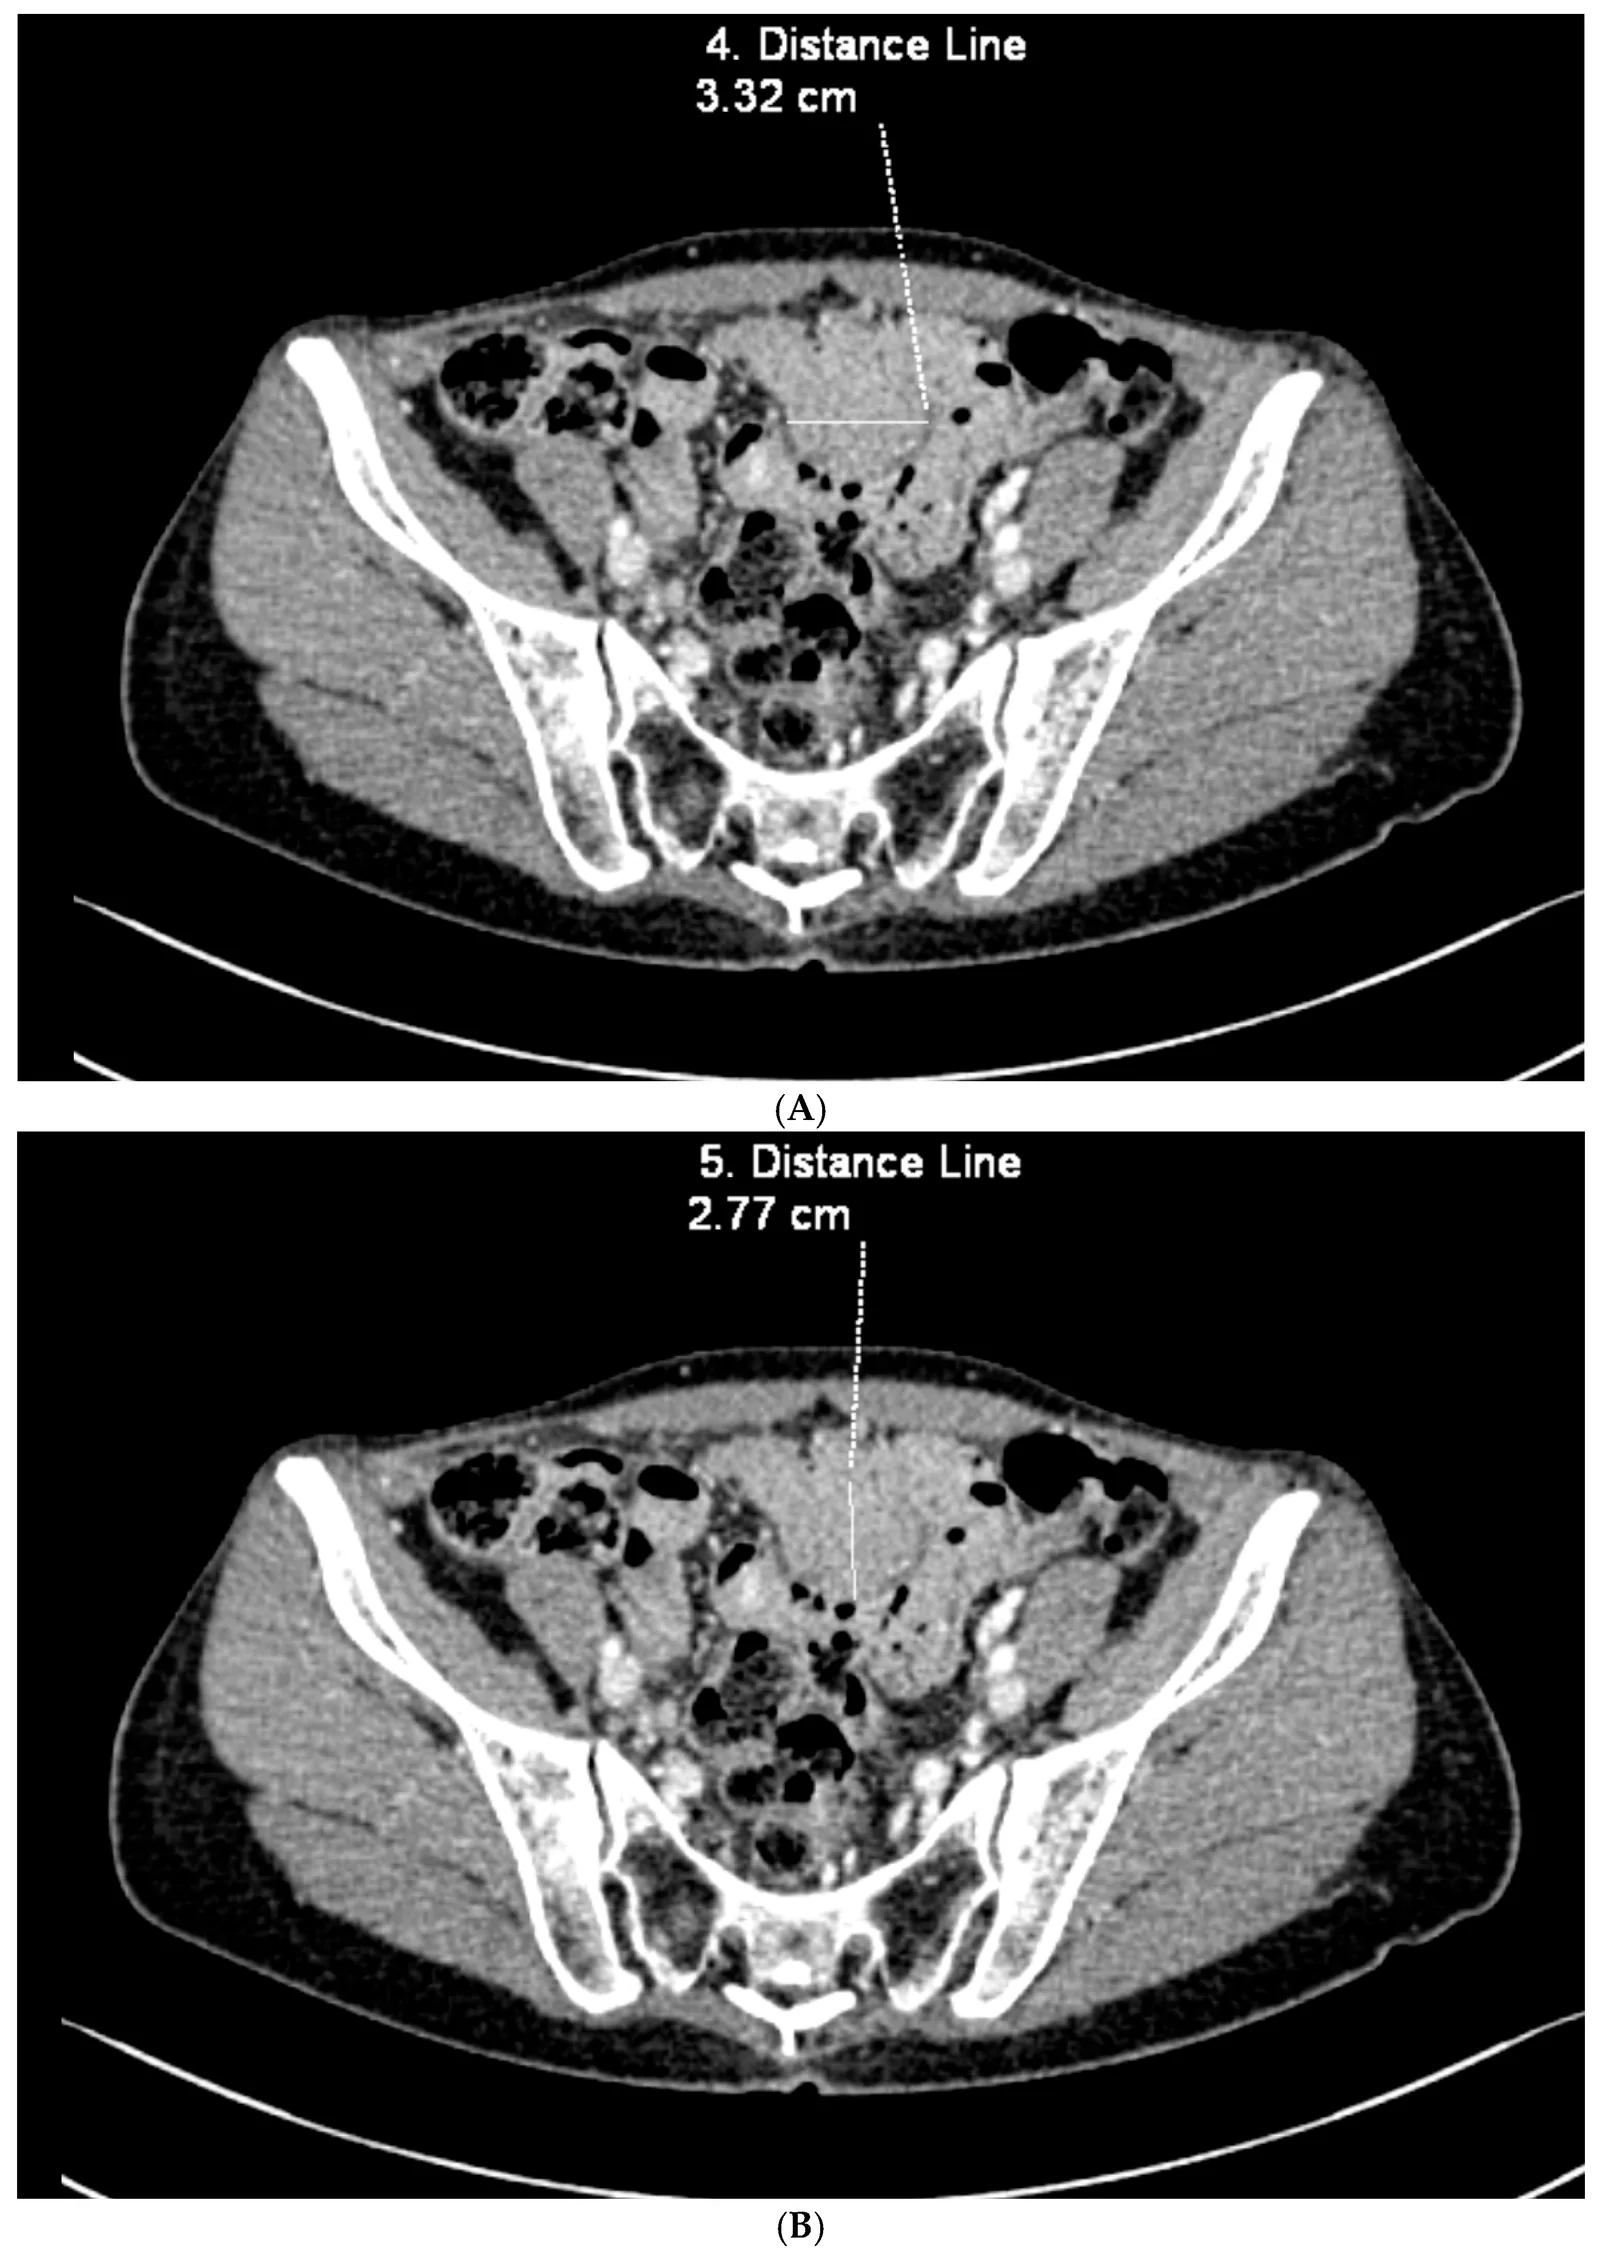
Contrast-enhanced computed tomography (CECT) of the abdomen and pelvis performed during the oncological work-up (11 September 2025). (A) Axial image (portal venous phase) demonstrating a pathologically enhancing tissue mass located superior to the uterine fundus, with a maximum transverse diameter of 3.32 cm (calliper). (B) Axial section showing the same adnexal lesion with a measured dimension of 2.77 cm (calliper).
